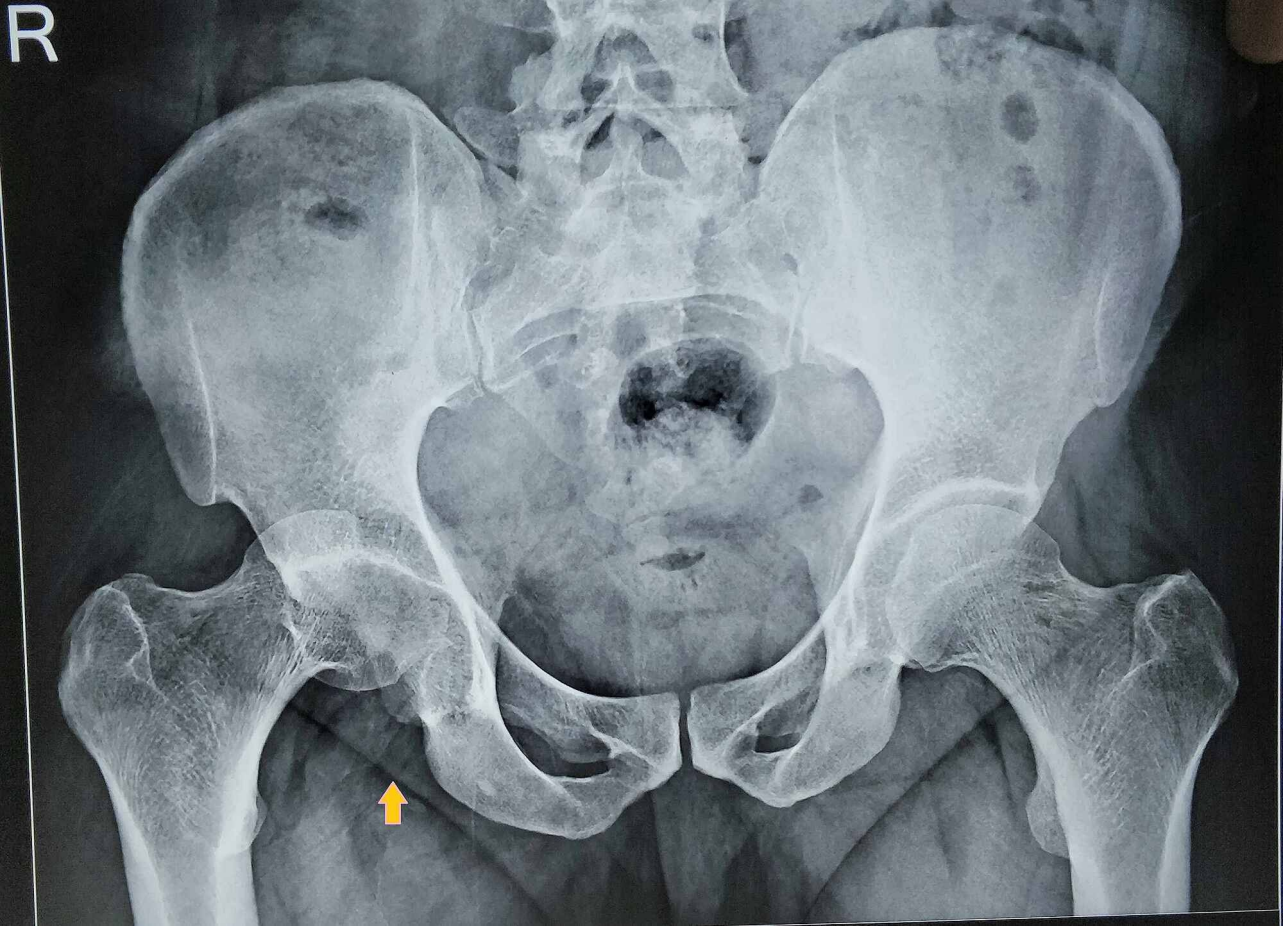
X-ray showing Pipkin fracture-dislocation (arrow)
